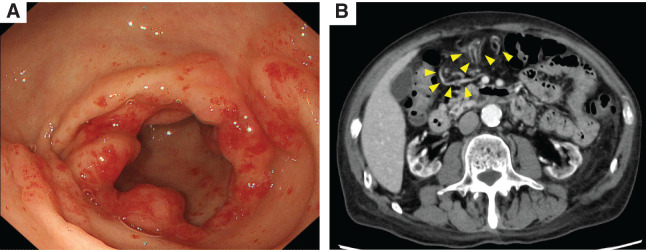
Endoscopic and contrast-enhanced CT findings before surgery. (A) EGD showing the characteristic “watermelon stomach” appearance, with prominent erythematous tortuous folds in the antrum, consistent with GAVE. (B) Contrast-enhanced CT demonstrating dilated omental arteries and veins. GAVE, gastric antral vascular ectasia; EGD, esophagogastroduodenoscopy
